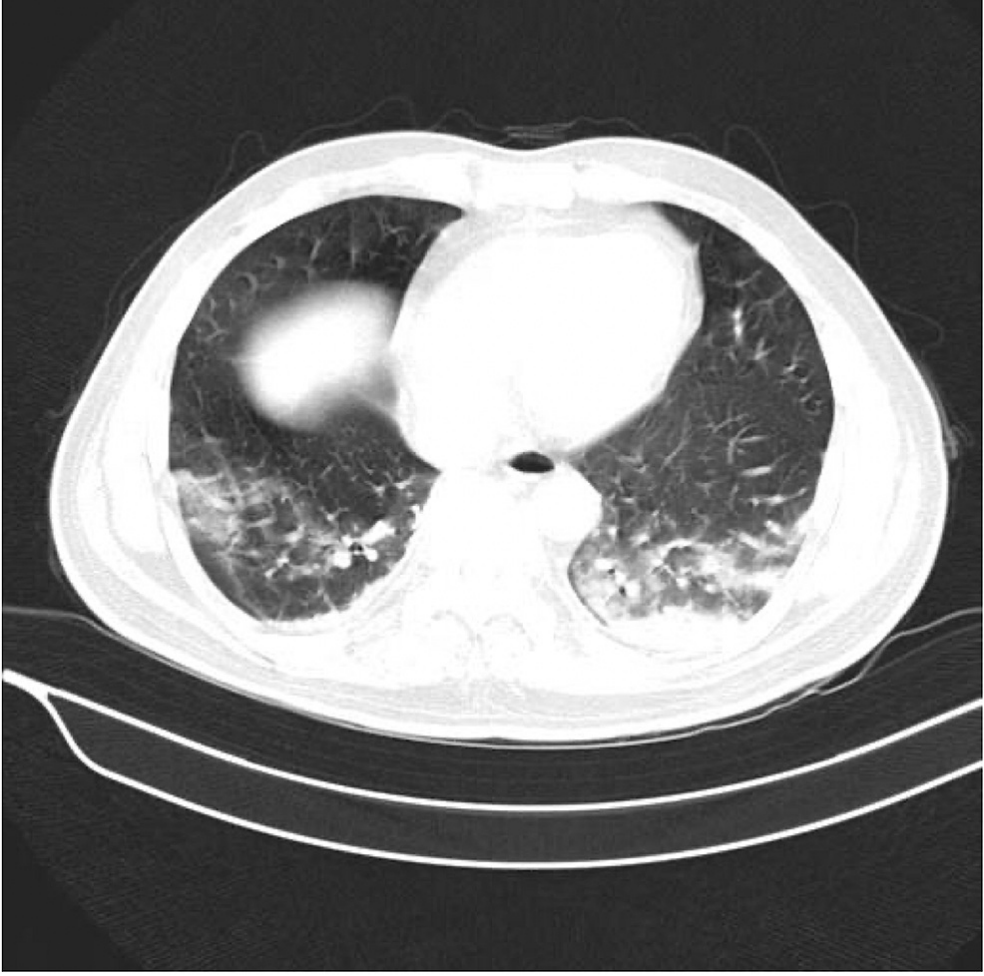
HRCT Thorax showing ground glass opacity suggestive of COVID-19
